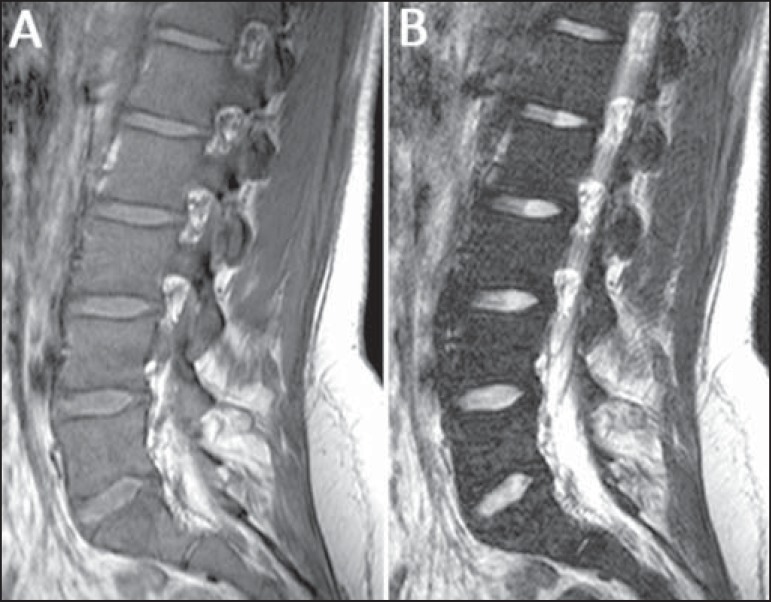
MRI of a female, 22-year-old patient recently diagnosed with Gaucher’s disease.
